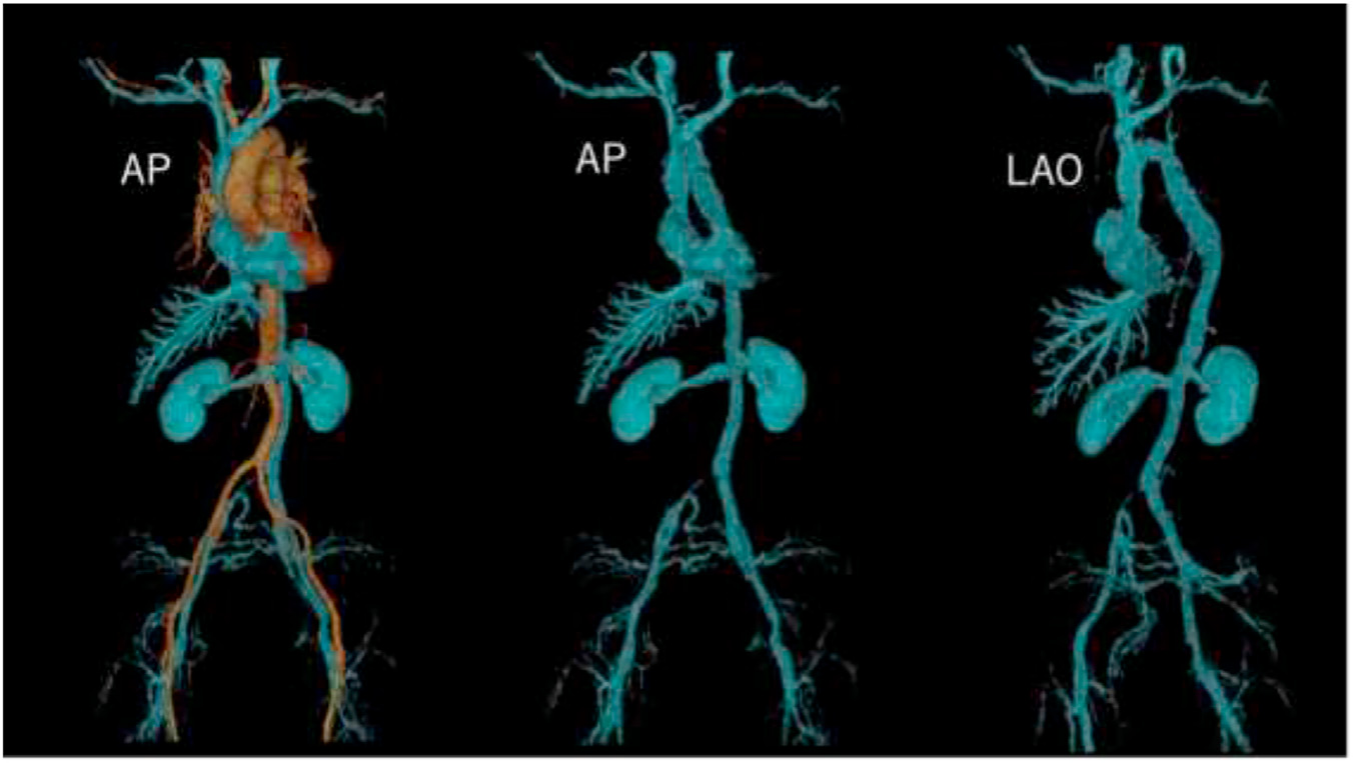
A postoperative 3D computed tomography scan shows that the infrahepatic segment of the inferior vena cava (IVC) is absent, and the infrarenal IVC begins with a developed azygos vein system, perfusing from the superior vena cava (SVC) to the right atrium. The hepatic veins are draining into the right atrium via the remaining thoracic IVC. The right common iliac vein is narrowed because of compression by the left common iliac artery. 3D = 3-dimensional; AP = anteroposterior; LAO = left anterior oblique.
